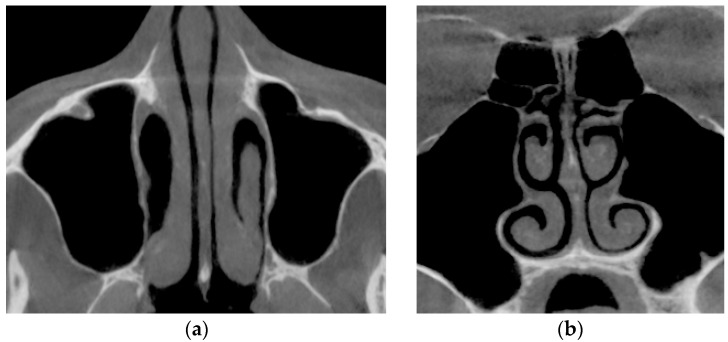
Computed tomography of a patient with the consequences of chronic rhinosinusitis: (a) axial tomographic section; (b) multiplanar reconstruction in frontal projection.
